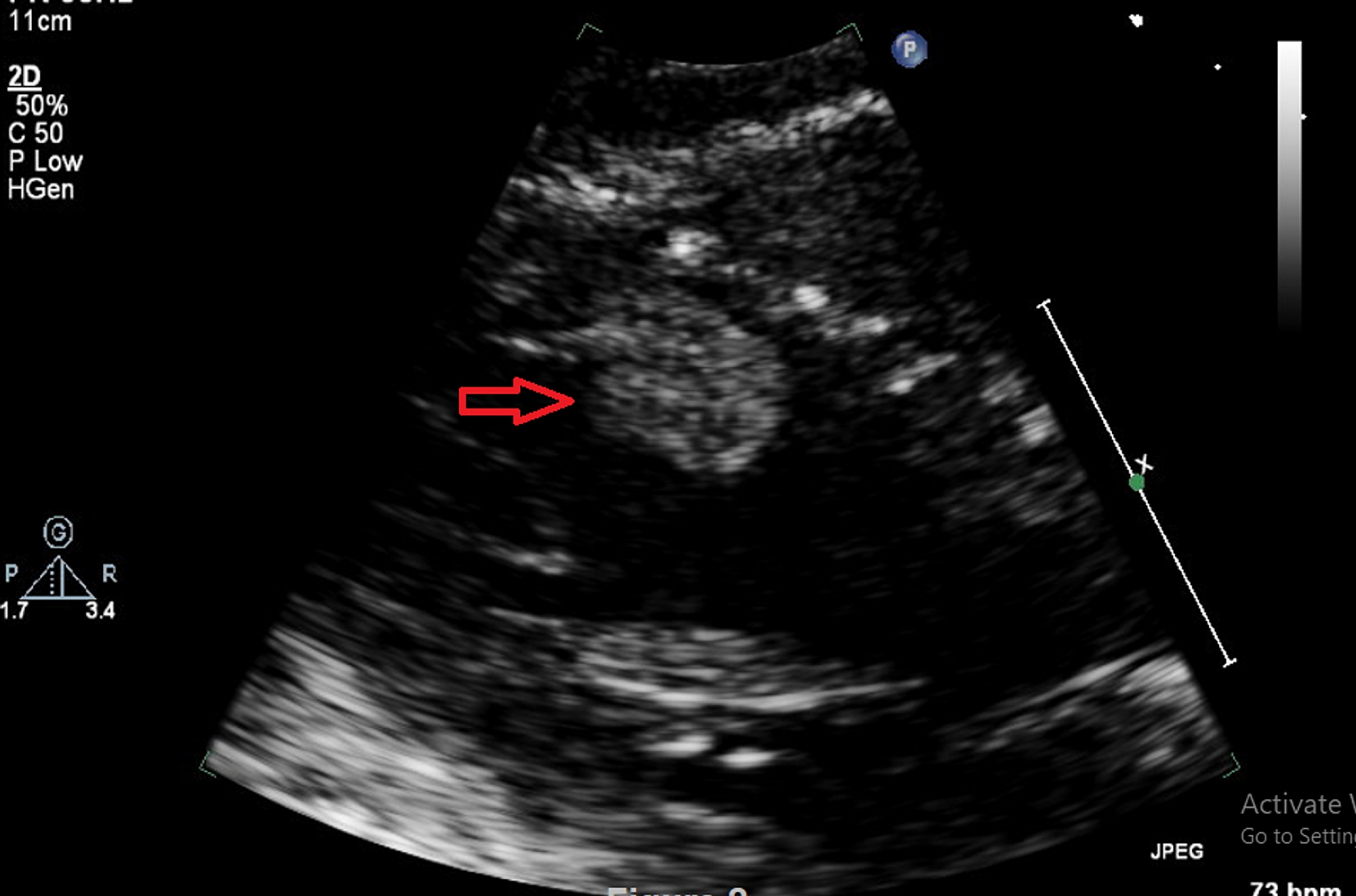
Transthoracic Echocardiography showing apical/septal mass in the LV (long axis, focused LV view)
